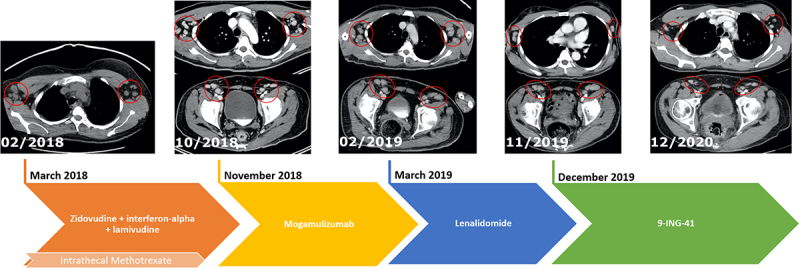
Timeline of Therapy with Correlating CT Imaging. (02/2018): Imaging at diagnosis showing enlarged bilateral axillary lymph nodes; (10/2018): progression of disease with increase in size of axillary and inguinal lymph nodes leading to transition to second-line mogamulizumab; (02/2019): Increase in size of axillary and inguinal lymph nodes leading to transition to third-line lenalidomide; (11/2019): persistently enlarged axillary and inguinal lymph nodes prior to start treatment with 9-ING-41, a selective small molecule inhibitor of GSK-3β; (12/2020): response with improvement of axillary and inguinal lymph nodes after 1 year of therapy with 9-ING-41.
